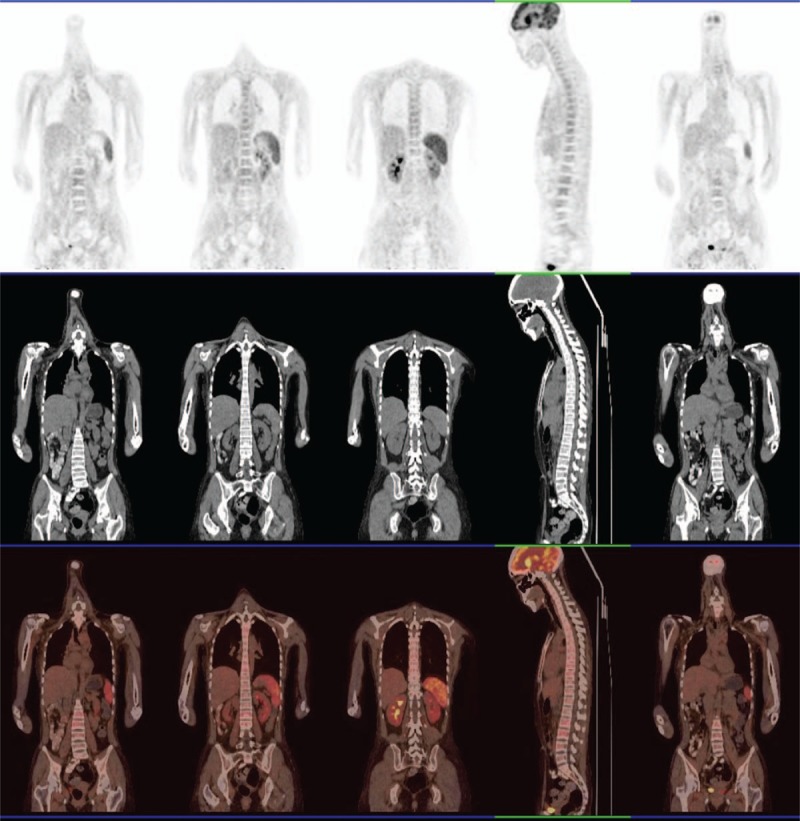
Malignant tumours and abscesses not found by PET/CT scan.
